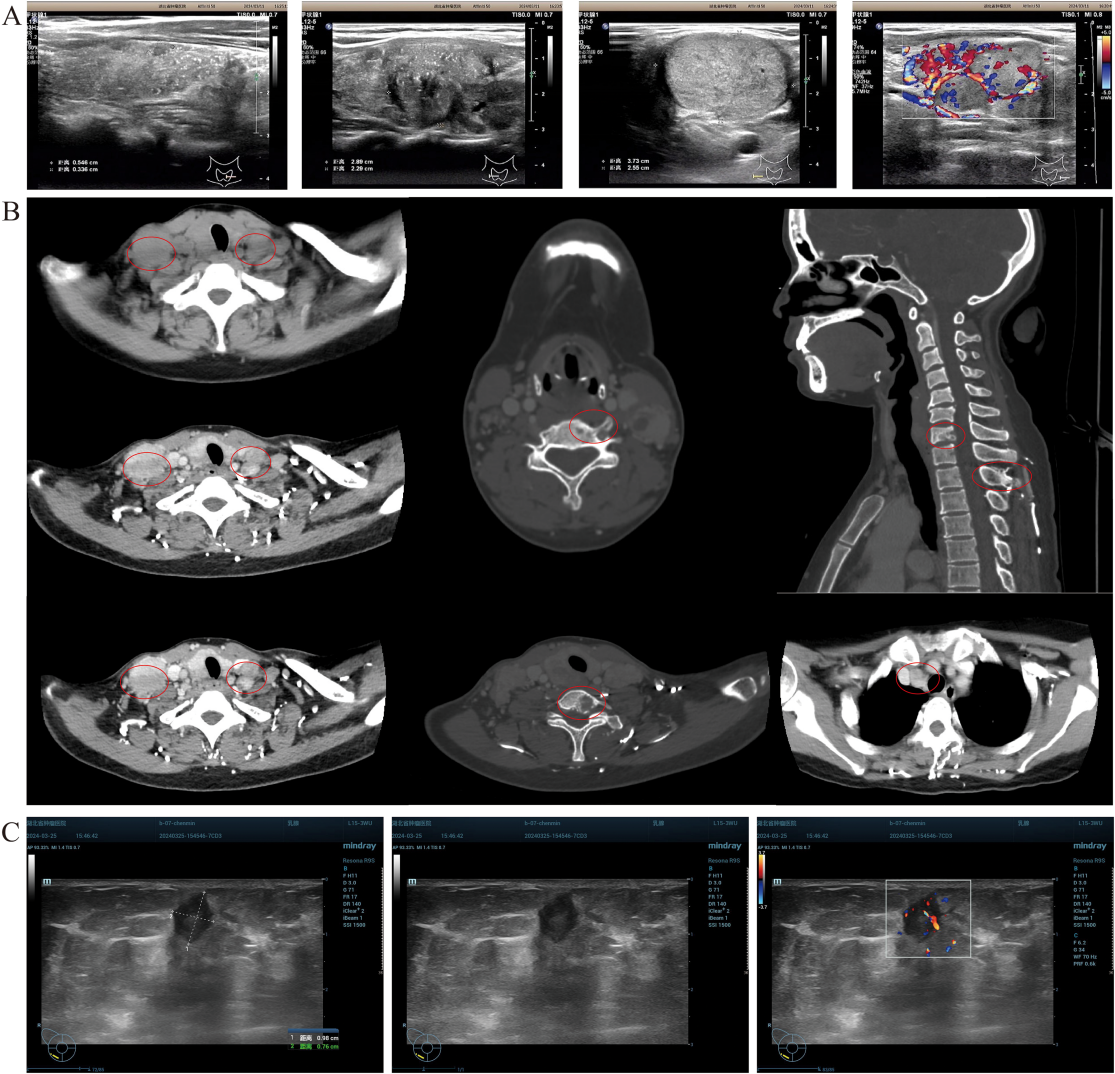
Patient imaging evaluation. (A) Thyroid color Doppler ultrasound revealed the presence of multiple punctate strong echoes within the bilateral lobes of the thyroid gland. These findings were accompanied by multiple hypoechoic and isoechoic masses, exhibiting indistinct boundaries and irregular contours. The internal echogenicity was heterogeneous, with evidence of multiple small calcifications. CDFI demonstrated discernible blood flow signals within these masses. Additionally, multiple lymph node echoes with well-defined boundaries but irregular shapes were identified in the bilateral cervical regions (zones II, III, IV, and VI). Some of these lymph nodes exhibited fusion, and the structure of the cortex and medulla was not clear. A subset of these lymph nodes displayed intense punctate echoes, and CDFI confirmed the presence of blood flow signals. (B) CT scan of the neck showed multiple lymph node metastasis in the anterior–superior mediastinum and multiple bone destruction in the lower cervical spine. (C) Breast color Doppler ultrasound examination of the right breast detected a 0.95 × 0.77 × 0.84 cm low-echoic mass, characterized by a clear boundary and an irregular shape. Certain sections of this mass exhibited angular features, with an aspect ratio > 1. CDFI analysis further revealed abundant blood flow signals within the mass.
